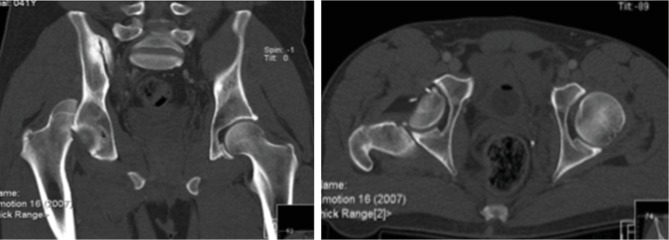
CT-scan of the pelvis showing a femoral head fracture associated with a posterior hip dislocation.
